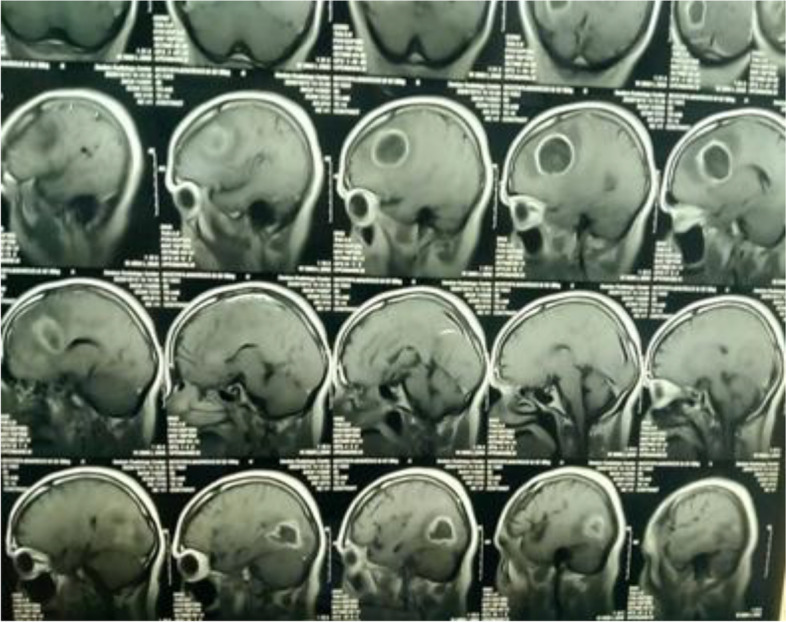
MRI with contrast, sagittal section showing ring-enhancing lesions in both frontal and parietal lobes
